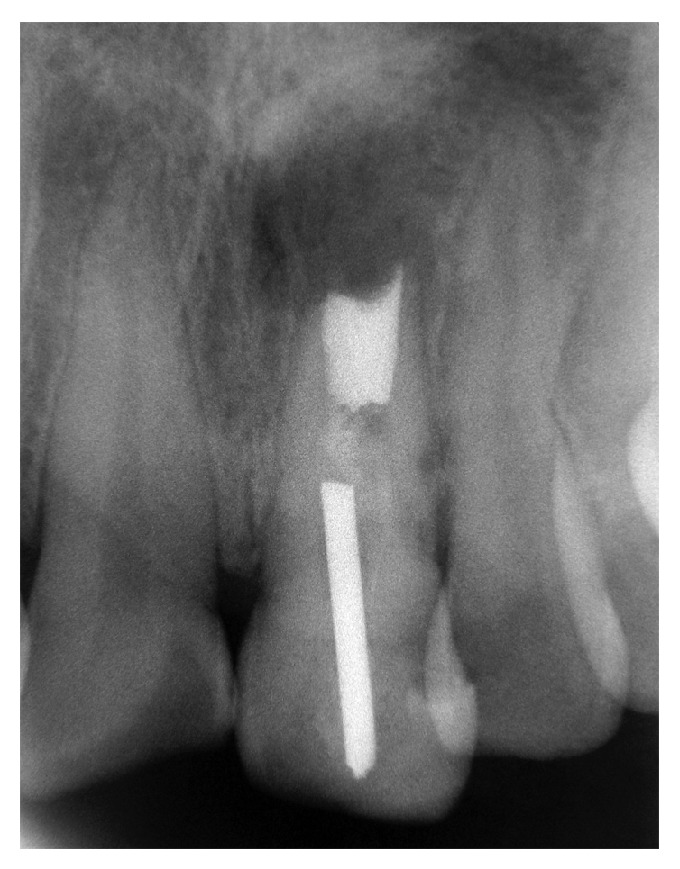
A postoperative radiograph shows the proper retrograde placement of thermoplasticized gutta-percha in direct contact with the resin located inside the main canal.
